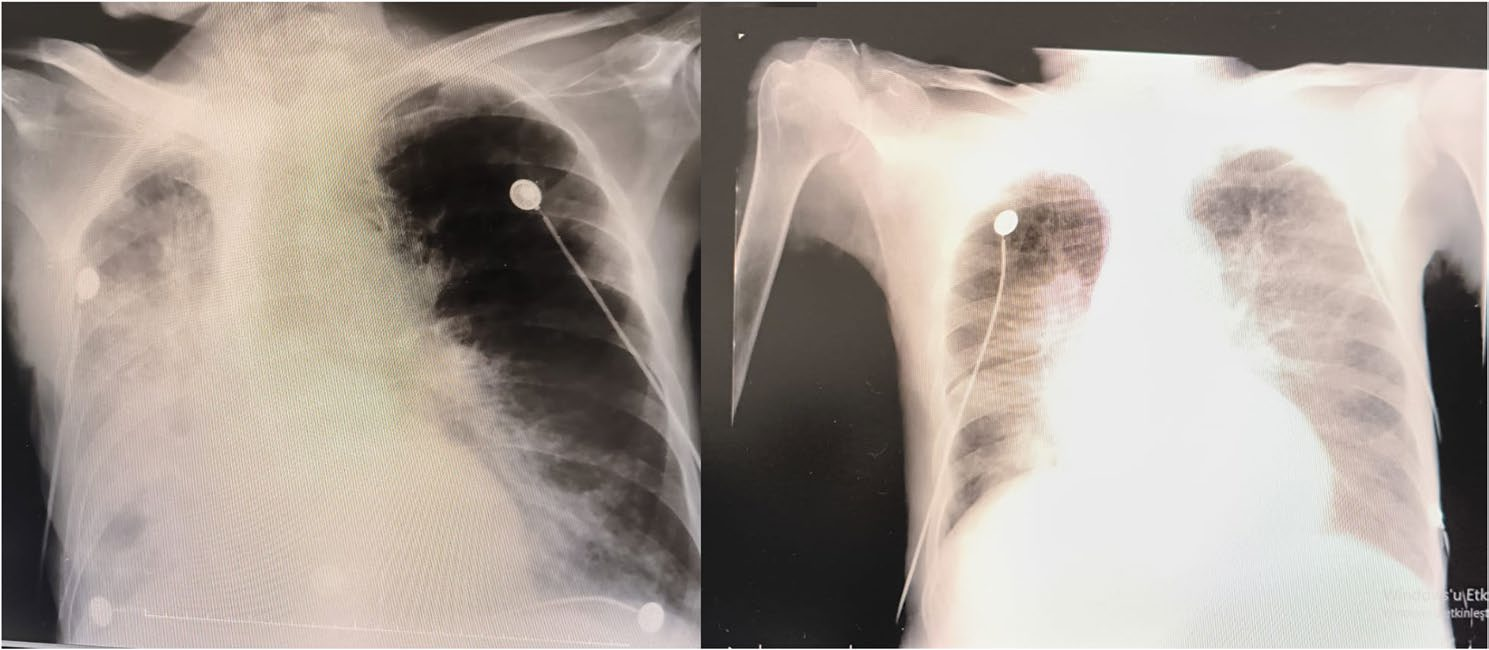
On the left, pleural effusion on the pa chest radiograph; on the right, pa chest radiograph after evacuating thoracentesis
